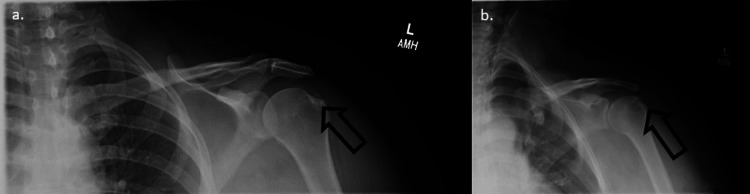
Radiographs of the left shoulder at eight week follow up demonstrating significant regression of calcific deposits. a) Anterior-posterior view. b) Internal rotation view.
